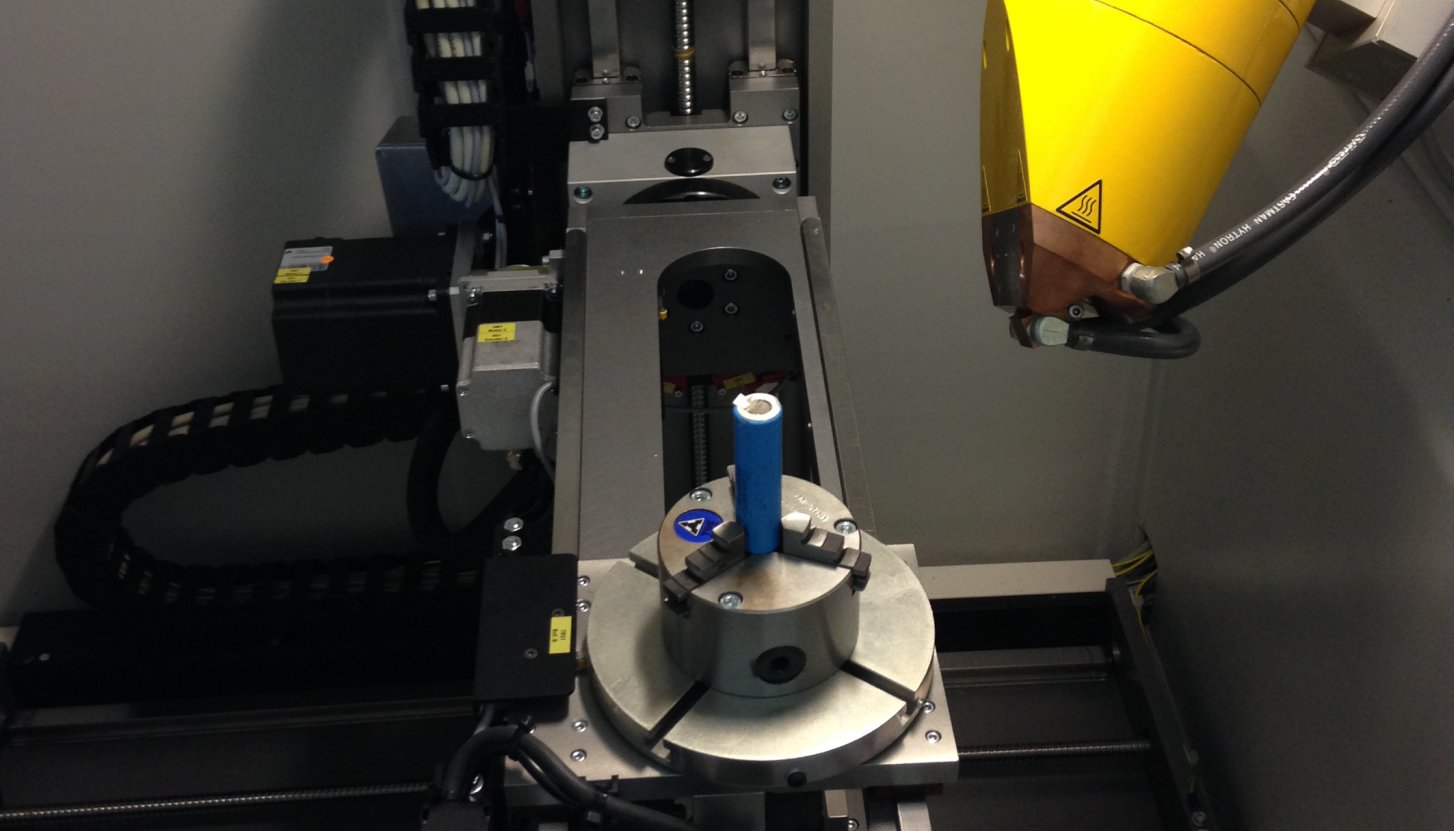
A micro-focused X-ray radiographic system at the University of Michigan.
